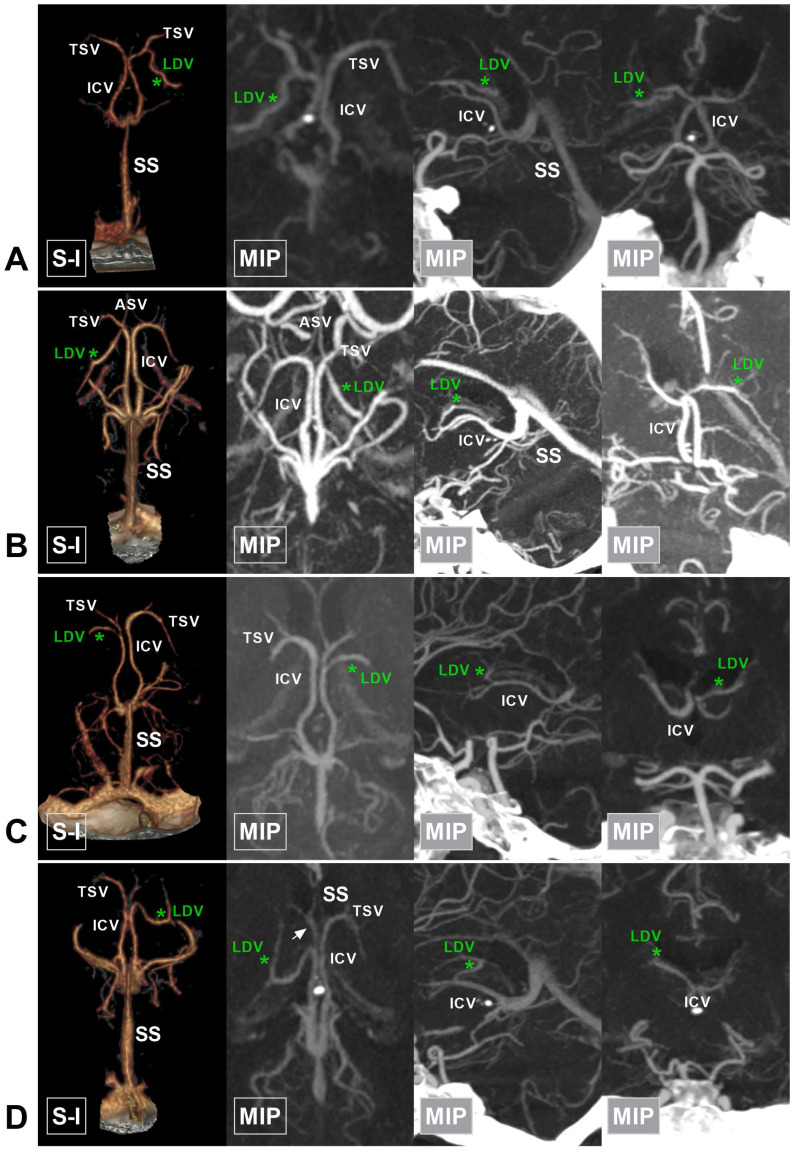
The anterior type of LDV. A, CTA and MIP show that the LDV (asterisk) drains into the TSV and courses posterolaterally. B, CTA and MIP show that the LDV (asterisk) drains into the confluence of the ASV and TSV. C, CTA and MIP show that the LDV (asterisk) drains into the ICV after the confluence of the ASV and TSV. D, CTA and MIP show that the LDV (asterisk) drains into the anterior half of the ICV (arrow). Abbreviations: ASV, anterior septal vein; CTA, computed tomography angiography; ICV, internal cerebral vein; LDV, lateral direct vein; MIP, maximum intensity projection; S-I, superior to inferior; SS, straight sinus; TSV, thalamostriate vein.
